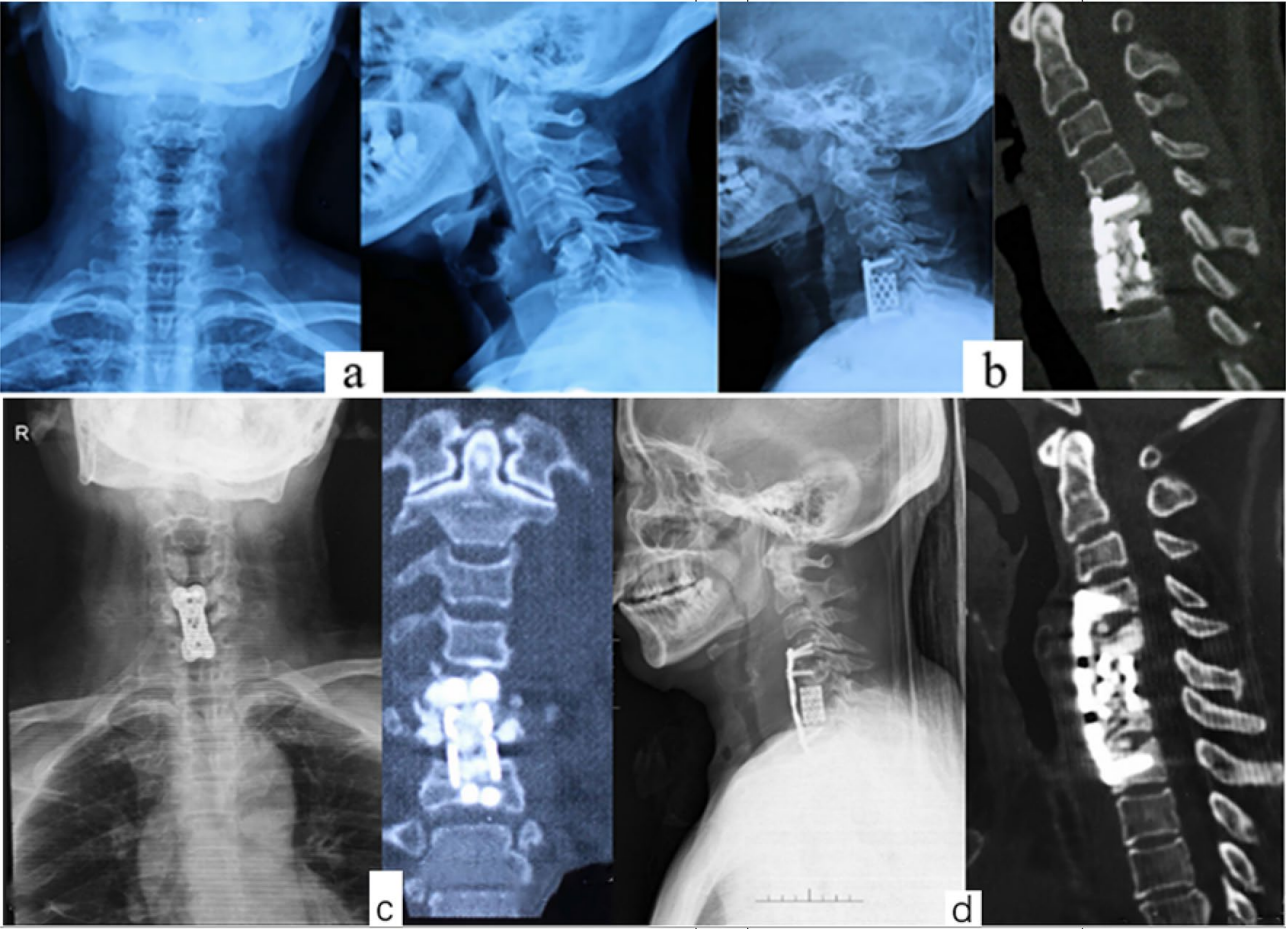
A 61 years old female with cervical TB at C5–7 and refractory kyphosis who didn’t undergo preoperative traction, (a) preoperative AP and lateral X-rays, no dynamic X-ray films because the patient was unable to flex or extend the neck. (b) Postoperative X-ray and CT, the disease lesion was cleared but the kyphosis was still there. (c) AP X-rays and CT-scan showing subsidence of titanium mesh, thus second operation was necessary to correct kyphosis and restore stability. (d) X-ray and CT-scan after the second operation. The kyphosis deformity was corrected.
